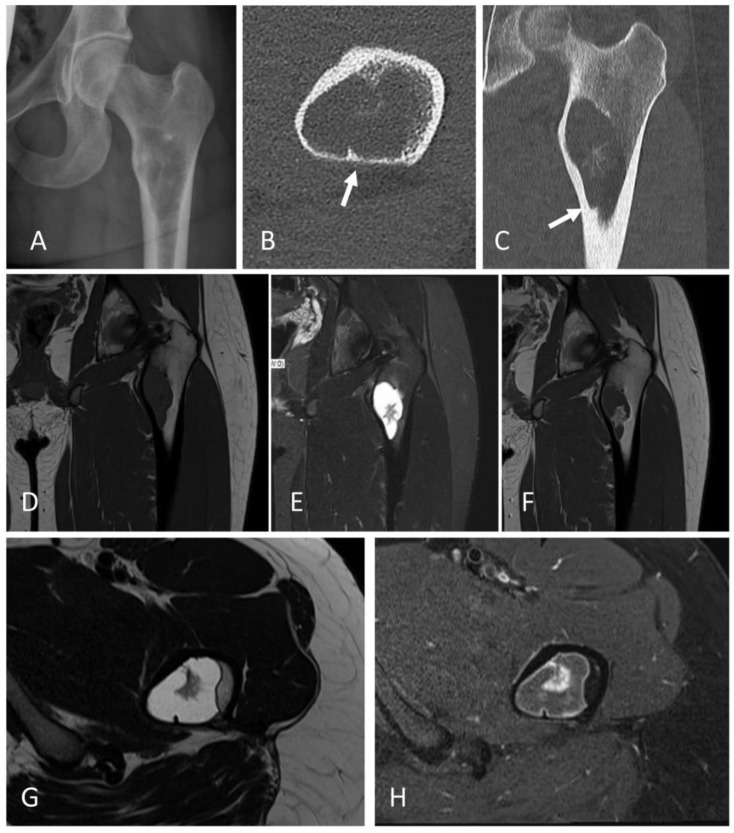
The 17-year-old boy with a lytic lesion of the proximal left femur (case #1) with a *FUS::NFATC2* fusion. (A) AP-radiograph; (B,C) axial and coronal CT-reformatted images show a hypodense lesion with focal central calcifications, thinning of the cortex (white arrows), and intralesional subseptations; (D–H) MRI shows that the tumor T1-weighted images have intermediate signal (D) and high signal on T2-weighted images (E,G) with enhancement of the cyst wall and irregular central subseptations (F,H). No bone marrow edema or periosteal reaction are present.
